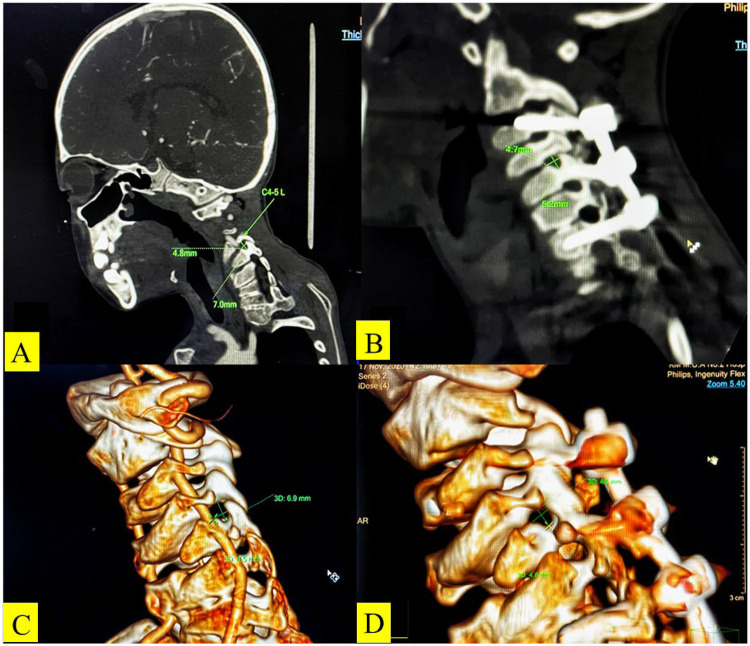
The vertical diameter of the right intervertebral foramen of C4-5 is 7.0 mm preoperatively (A) and 5.2 mm postoperatively (B) on the coronal CT images. Three-dimensional CT images reveal that the vertical diameter of the right intervertebral foramen of C4–5 is 6.9 mm preoperatively (C) and 4.6 mm postoperatively (D).
